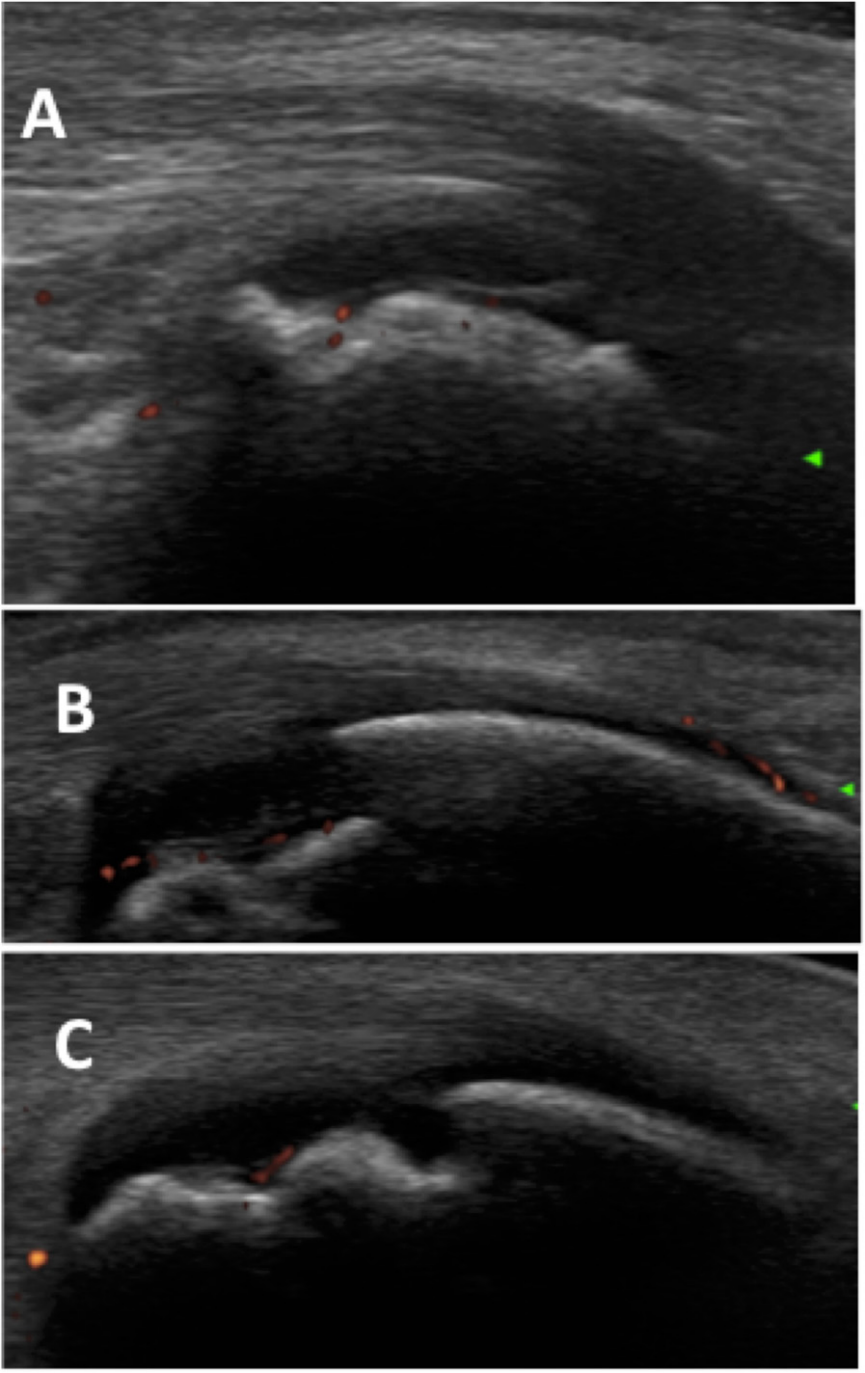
The calcaneus bone appears fragmented in children respectively aged 6 years (a), 8 years (b), and 10 years (c). Feeding vessels can be detected by power Doppler (*red* dots)
